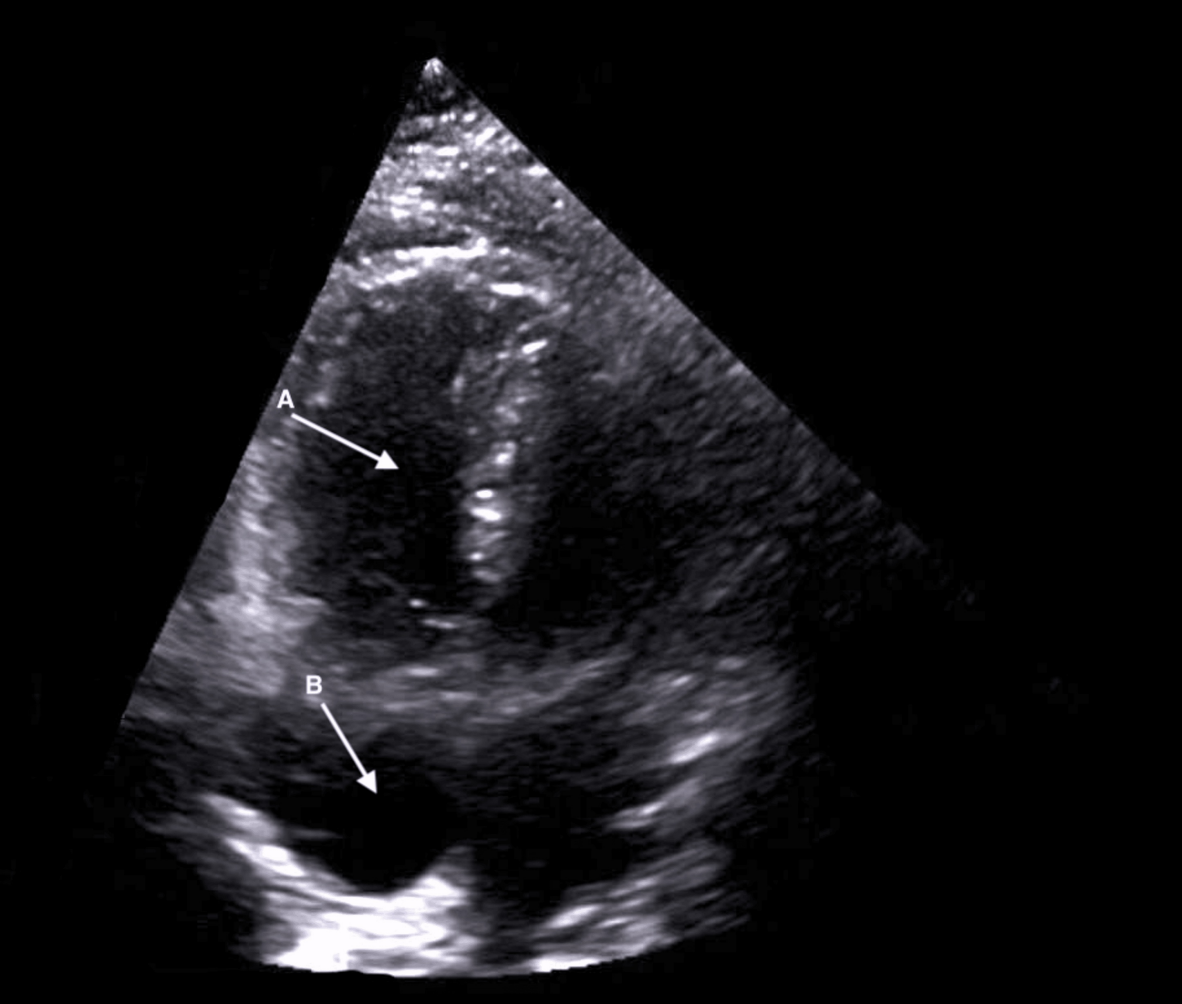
POCUS in the apical four-chamber view performed during resuscitation, showing right ventricular (arrow A) and right atrial (arrow B) dilatation POCUS: point-of-care ultrasound
